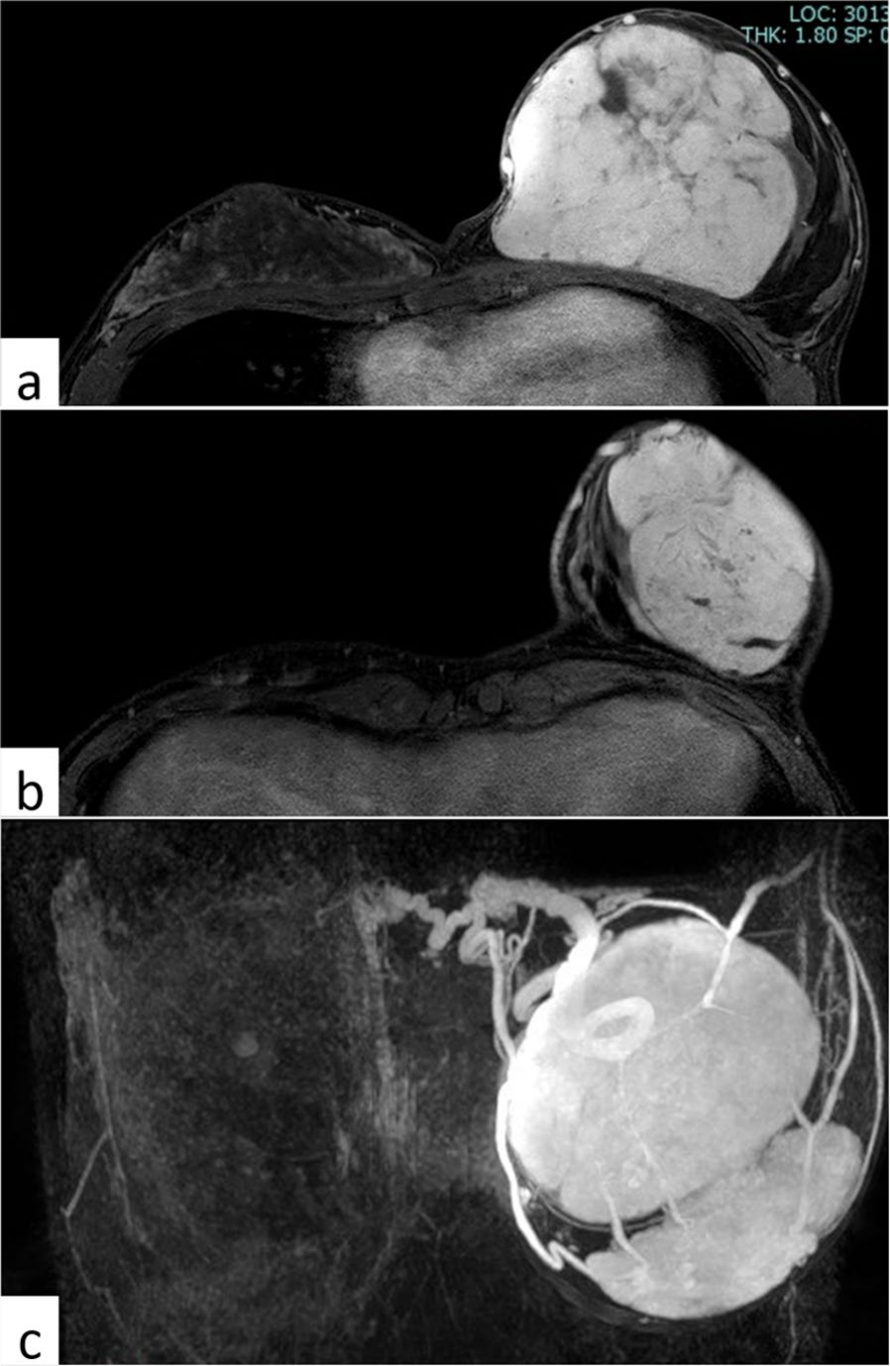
Contrast-enhanced magnetic resonance imaging. a Well-defined 10 cm mass occupying the upper part of the left breast and b 7.7 cm mass in the lower-outer part of the left breast. c The two masses were fed by the lateral thoracic and internal thoracic arteries and the drainage veins were remarkably dilated
